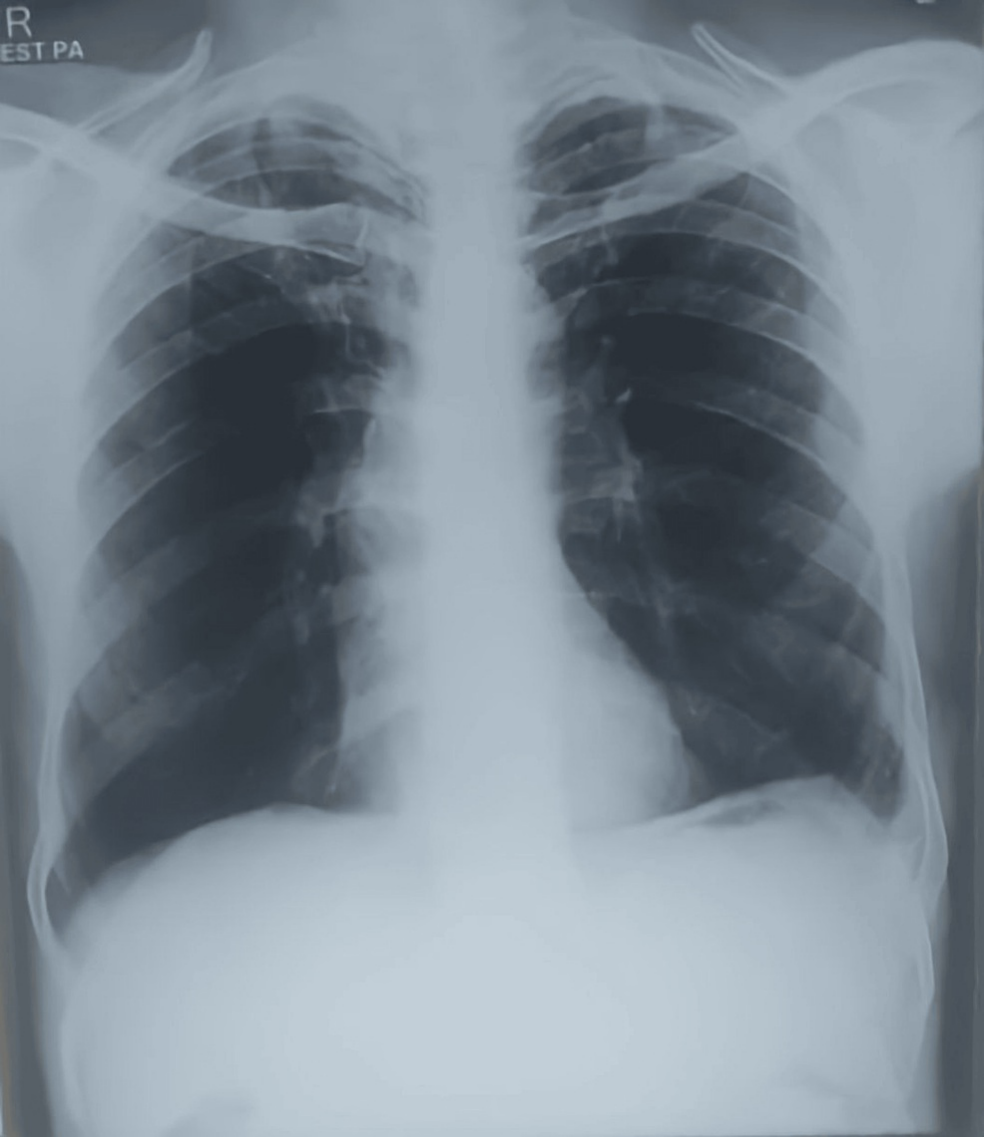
Follow-up chest X-ray after six months showing good clearance of empyema.
